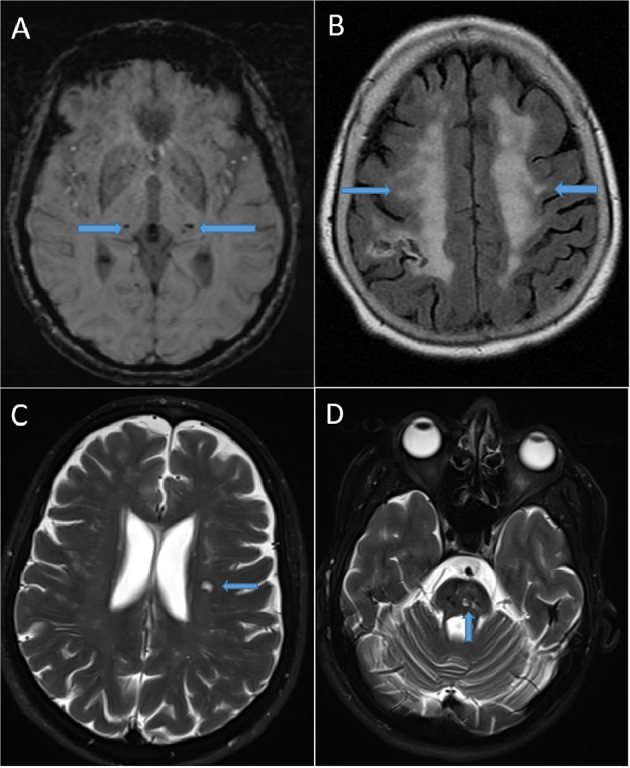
MRI examples of SVD features in Fabry Disease patient cohort. (A) Axial susceptibility weighted image shows the presence of bilateral thalamic microbleeds for an SVD microbleed score of 1 (microbleeds present) in 60-year-old male patient. (B) Axial FLAIR shows confluent subcortical white matter disease for a Fazekas score of 3 in 81-year-old female. A chronic right parietal infarction involving a portion of the right MCA territory is also partially imaged. (C) Axial T2 fat saturated image shows a pontine lacunar infarction in 64-year-old female. (D) Axial T2 fat saturated image in the same patient as image C shows another lacunar infarction in the left corona radiata. An additional lacunar infarction of the genu of the corpus callosum in this patient (not shown) results in an SVD lacunar score of 2 (3–5 lacunes).
